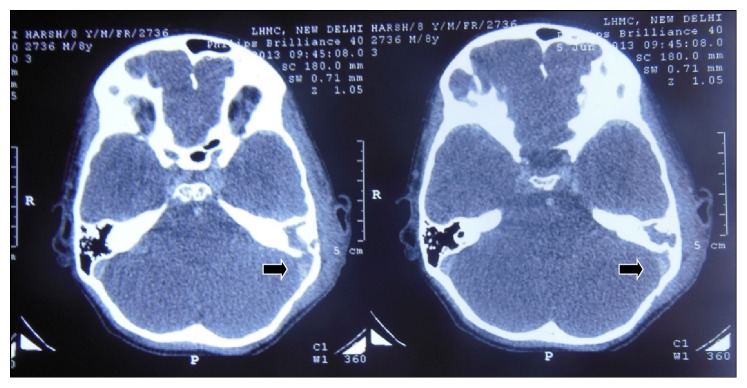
Contrast-Enhanced CT scan showing lateral sinus thrombosis (arrow).
